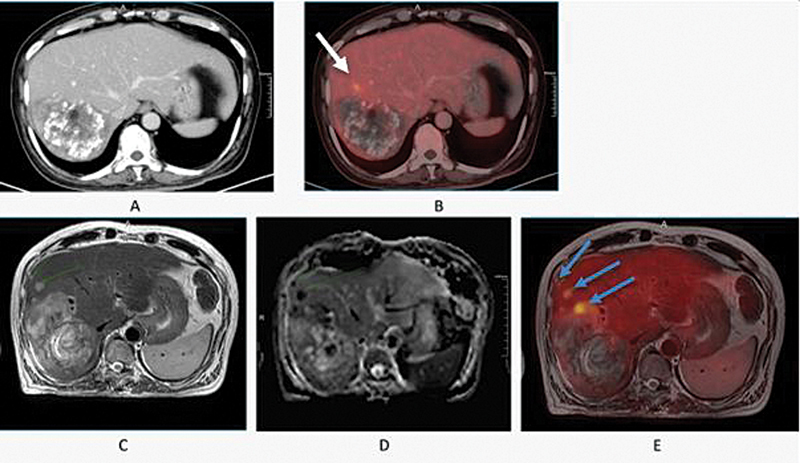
A 66-year-old man with hepatocellular carcinoma, showing a large hepatic mass with necrosis, radio-dense foci (post-TARE status), heterogeneous peripheral enhancement, and adjacent mild FDG uptake anteriorly as can be seen in PET/CT images (
